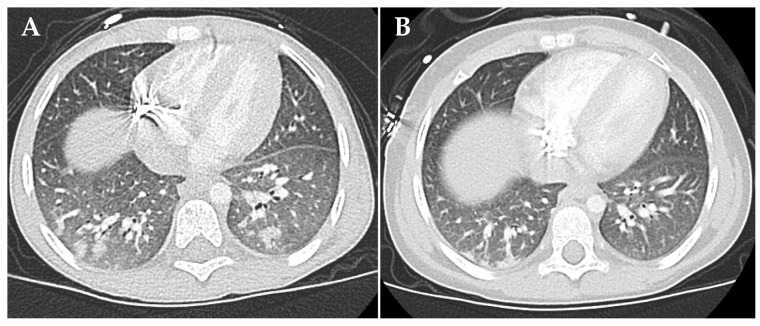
High-resolution computed tomography findings of busulfan-induced lung injury in patient 2 at diagnosis (A) and six months after start of the anti-inflammatory therapy (B). (A) Shown are atypical pneumonic changes, characterized by ground-glass opacification and patchy consolidation in the lower lobes. (B) Shown is the regression of consolidations and opacities. There is no evidence of pulmonary fibrosis.
